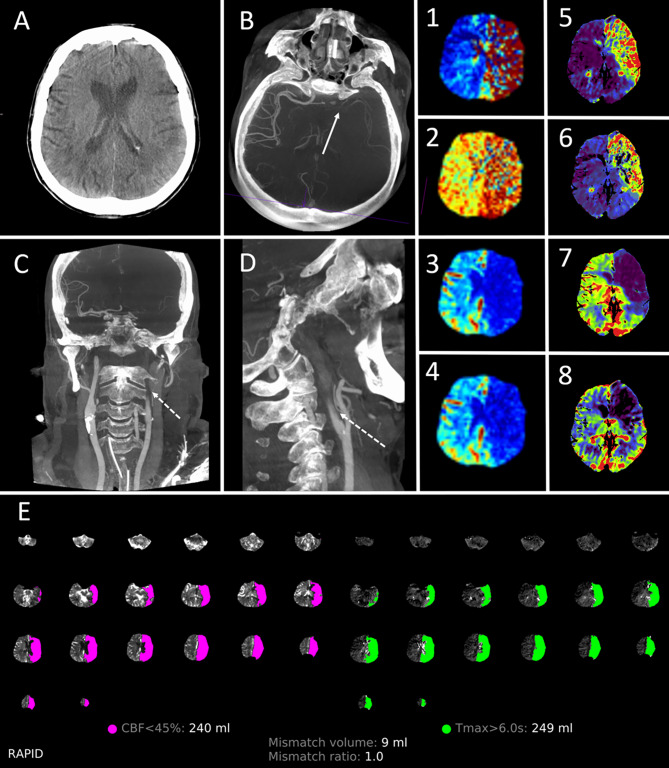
Exemplary images processed by the new multimodal FD-CT approach. 79-year-old female with an occlusion of the Carotid-T left, NIHSS 16. NE-FDCT showed no demarked infarct (A). FD-CTA reveals absent filling of the left MCA-territory with almost no collateralization (B; continuous arrow) due to an occlusion of the proximal ICA on the left (C and D; dashed arrows). Color-coded maps of RAPID ANGIO (1–4) and of the Siemens perfusion prototype software (5–8) show a severe prolongation for Tmax (1) and TTP (5), MTT (2 and 6) and a significantly reduced CBF (3 and 7) and CBV (4 and 8). Brain hemodynamics were severely impaired, as the automated perfusion analysis using RAPID ANGIO showed a poor mismatch ratio of 1.0 (E). The ratio was calculated by the division of the potential salvageable tissue, estimated by the delayed Tmax (green), and the presumed infarcted brain, represented by the CBF reduction (pink)
